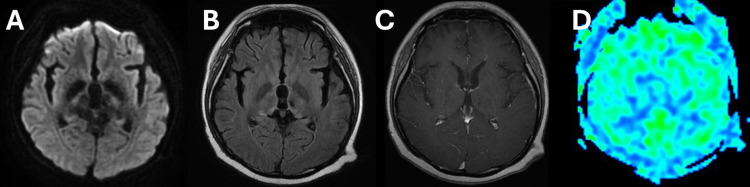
Post-treatment magnetic resonance imaging (MRI) findings. A) A diffusion-weighted imaging sequence and B) a fluid-attenuated inversion recovery image revealed that the high-signal intensity areas had dramatically shrunk with C) no contrast enhancement on a gadolinium T1-weighted image, and D) the high-perfusion sites on arterial spin-labeling MRI had diminished.
